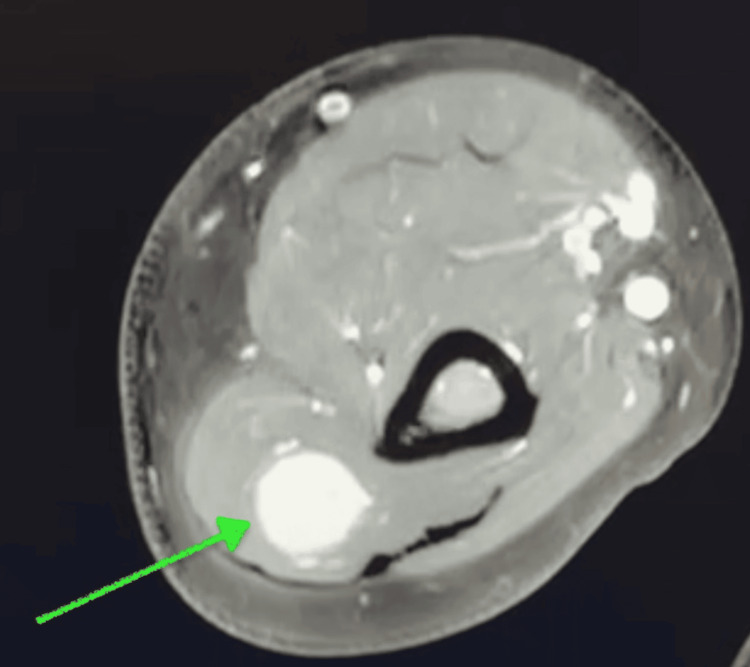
Axial view of a contrast-enhanced MRI of the right arm showing a peripheral nerve tumor (green arrow) deep to the triceps posterior fascia along the lateral head of the triceps
